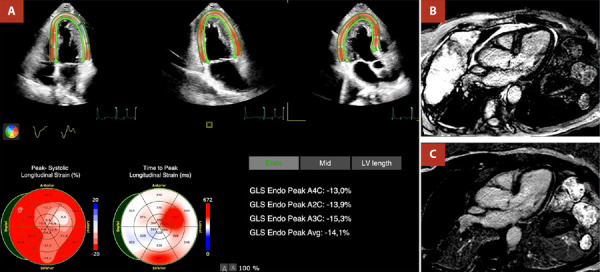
Transthoracic echocardiogram and cardiac magnetic resonance in a patient with ICI cardiotoxicity. A) Transthoracic echocardiogram revealing decreased left ventricular global longitudinal strain (-14.1%). B) and C) Cardiac magnetic resonance in T1-weighted inversion-recovery sequence demonstrating mid anteroseptal late gadolinium enhancement, consistent with myocarditis.
